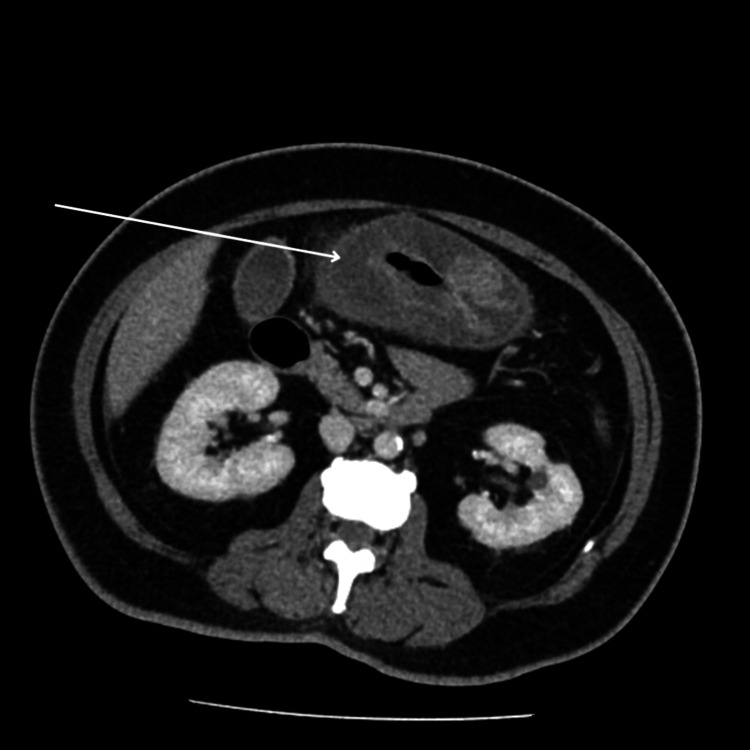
CT images detailing diffuse thickening of the gastric wall (white arrow).
